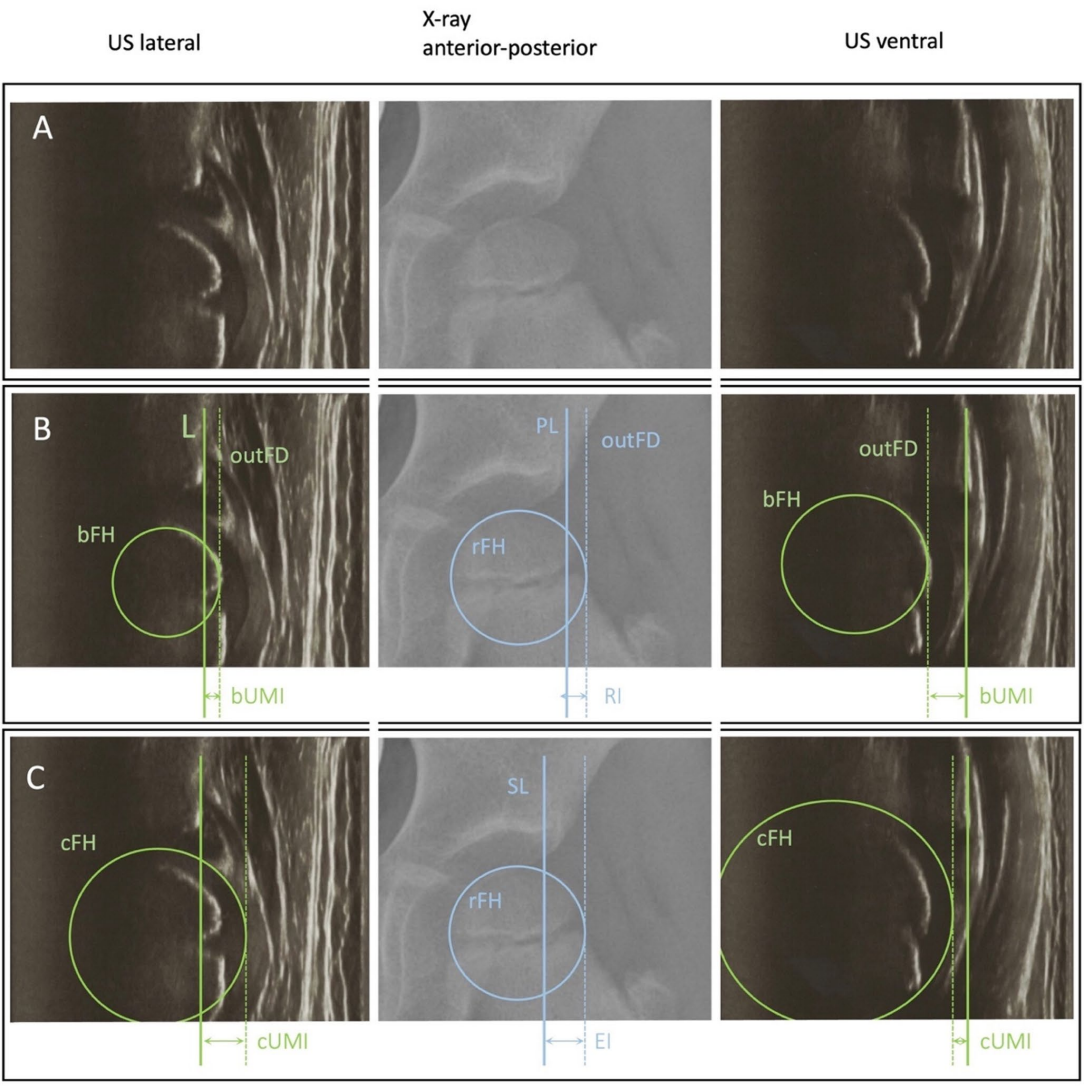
Exemplary US imaging. Left row: US lateral view; middle row: x-ray a.p. view; right row: US ventral view. (A) Native images. (B) Markers depict borders using the bony femoral head for calculating the bUMI and the radiographic RI. (C) Markers depict borders using the cartilaginous femoral head for calculating the cUMI; L = line through the US bony lateral edge of the acetabulum, bFH = extrapolated bony femoral head using US, cFH = extrapolated cartilaginous femoral head using US, rFH = extrapolated bony femoral head using radiography, PL = Perkins Line at the most lateral edge of the acetabulum, SL = vertical line using the lateral edge of the sourcil. dotted line = outFD (line situated at the outer femoral diameter)
